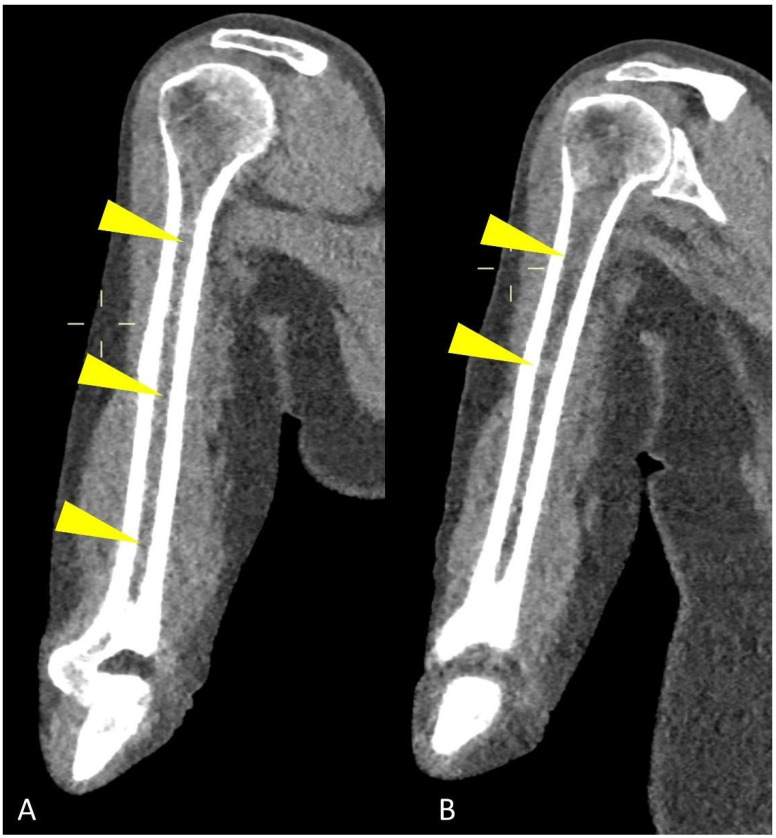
Coronal CT image showing (yellow arrowheads) humeral abnormal medullary lesions with high CT density in a patient with MM with (A) diffuse and (B) focal pattern.
